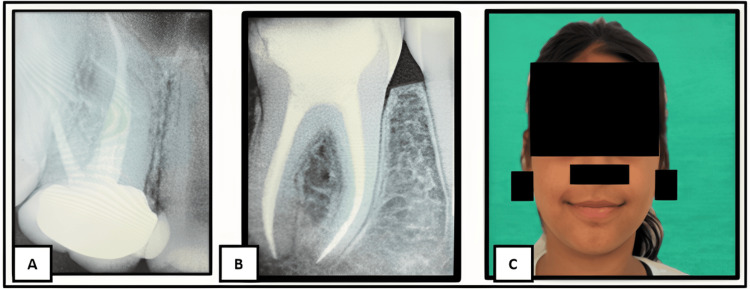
Postoperative radiographs of teeth 16 and 46 with resolution of extraoral swelling (A) Postoperative radiograph of tooth 16. (B) Postoperative radiograph of tooth 46. (C) Postoperative extraoral photograph.
